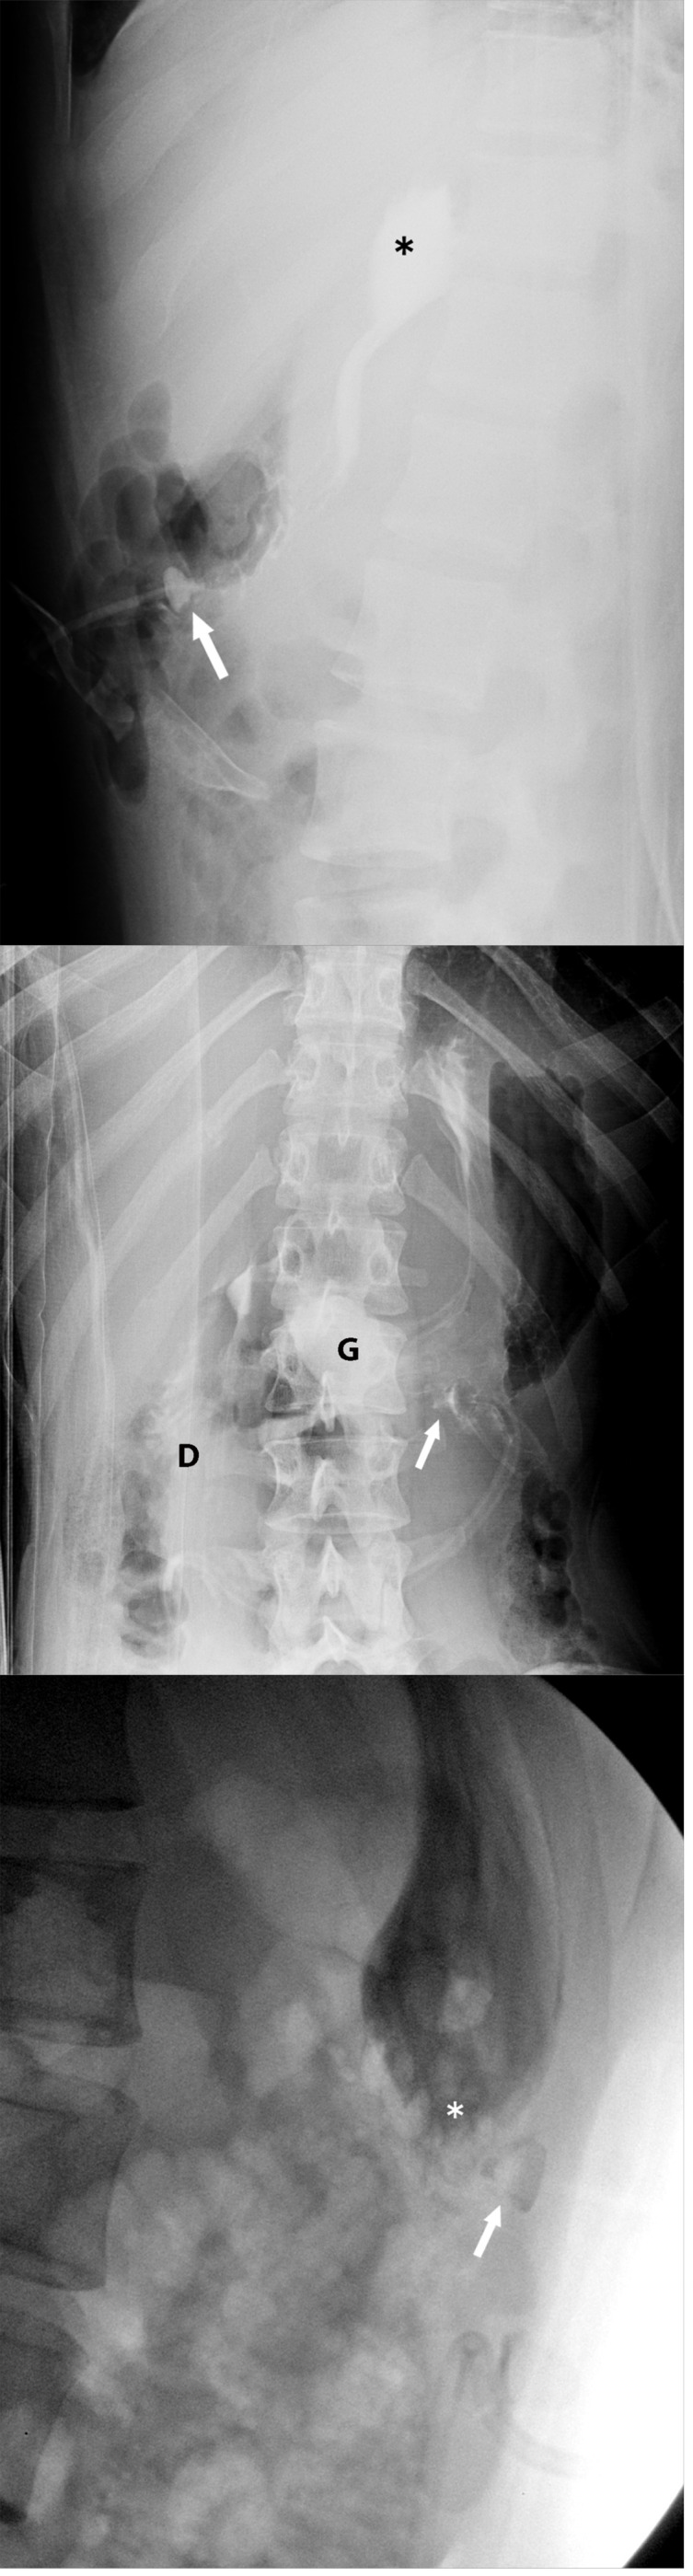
17-year-old female with indeterminate abdomen radiograph exam and high clinical suspicion who underwent a fluoroscopic G-tube contrast check exam confirming a true positive (abnormal exam). a Cross-table lateral abdomen radiograph shows intraluminal contrast flow into the gastric fundus (*). Alignment of the G-tube retention component (arrow) within the gastric lumen is indeterminate. b Right lateral decubitus abdomen radiograph shows definitive intraluminal contrast flow into the gastric lumen (G) and duodenum (D). Alignment of the G-tube retention component (arrow) within the gastric lumen is uncertain. c Left posterior oblique view during fluoroscopic G-tube contrast check shows the G-tube retention component (arrow) outside of the gastric luminal contrast (*). d Right posterior oblique fluoroscopic view (tangential view to Fig. 8) confirms extraluminal alignment of the G-tube retention component (arrow) relative to the gastric luminal contrast (*) administered under fluoroscopy.
